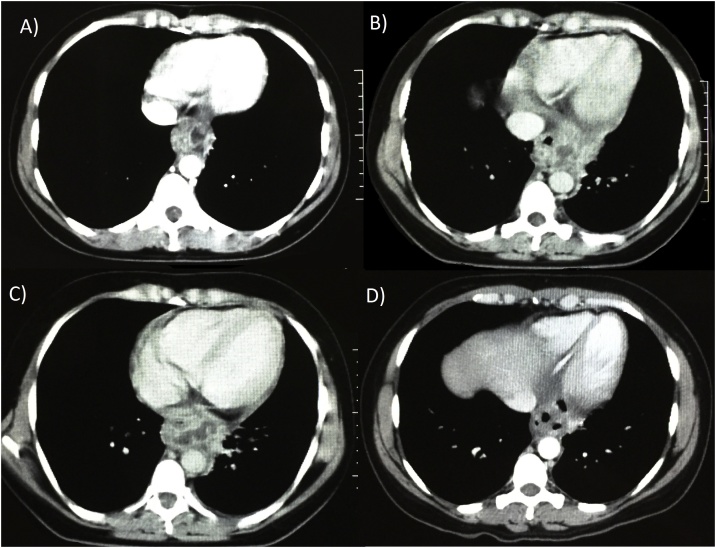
Computed tomography. Along two-year follow-up, the patient presented four (A–D) episodes of mediastinal abscesses.
